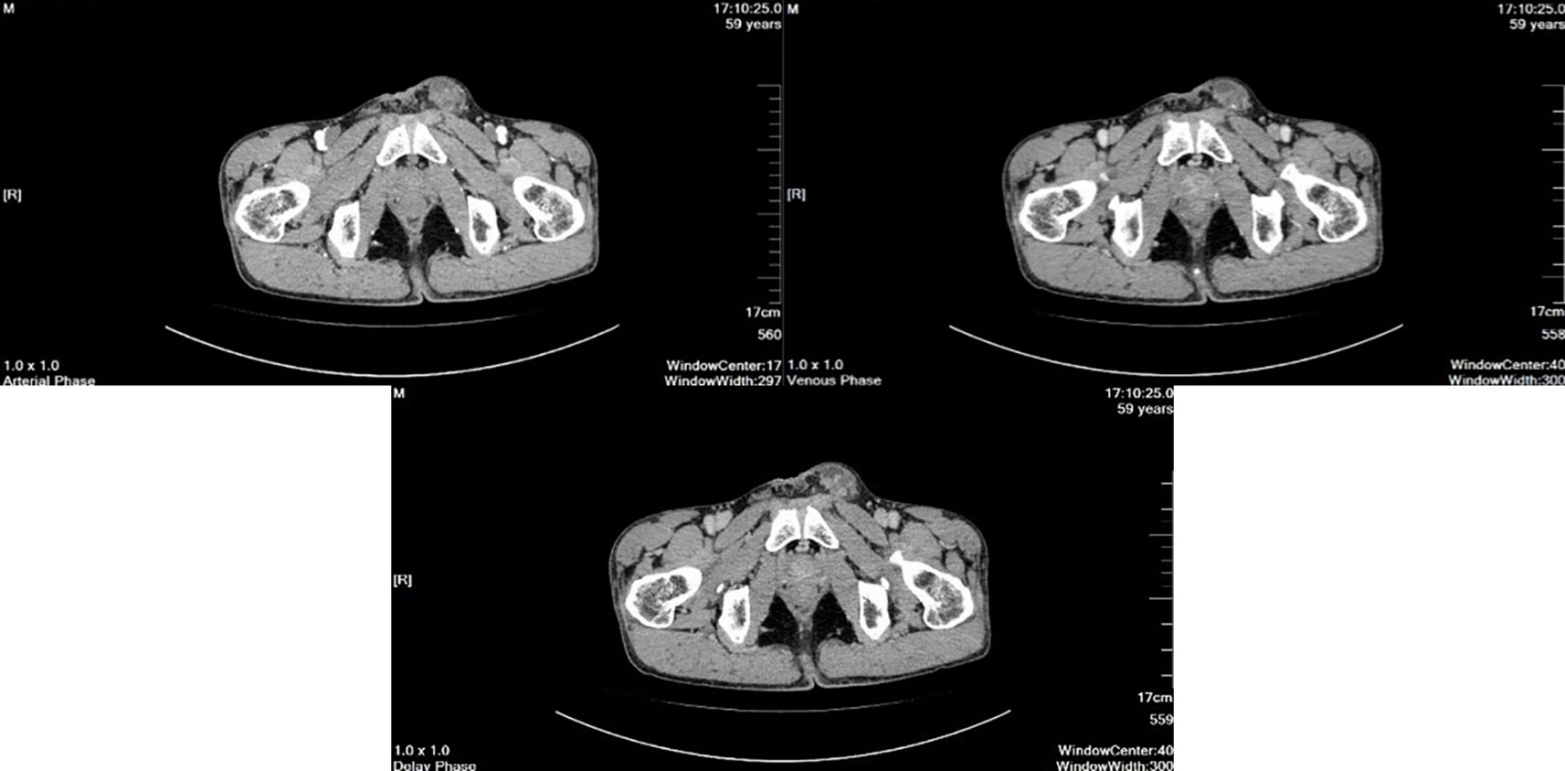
The enhanced computed tomography (CT).
